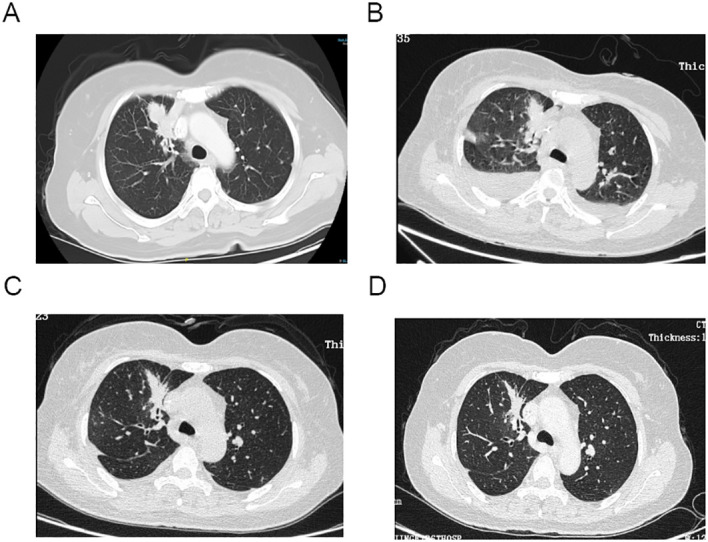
Chest CT images. (A) Former chest CT image. (B-D) Chest CT images of the first hospital admission, second hospital admission, and the outpatient follow-up, respectively.
